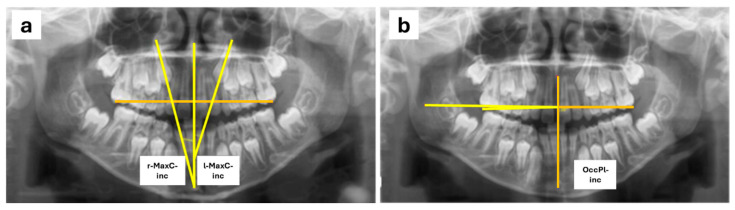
Radiographic measurements conducted in orthopantomographies: MaxC-inc (a) and OcclPl-inc (b).
